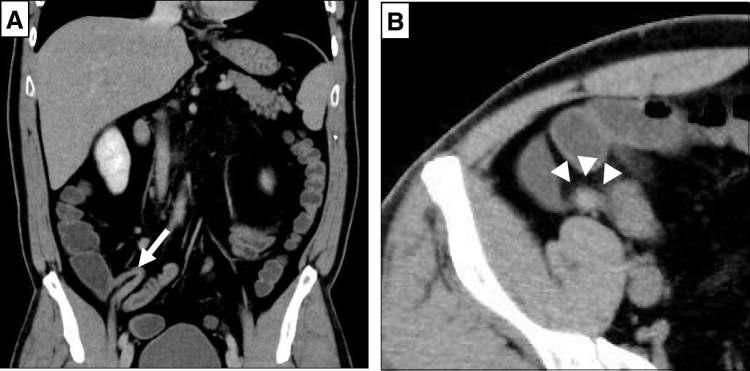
Contrast-enhanced CT. (A) The appendix appeared enlarged (white arrow). (B) A hyperdense area was identified within the appendiceal lumen (white arrowheads).
